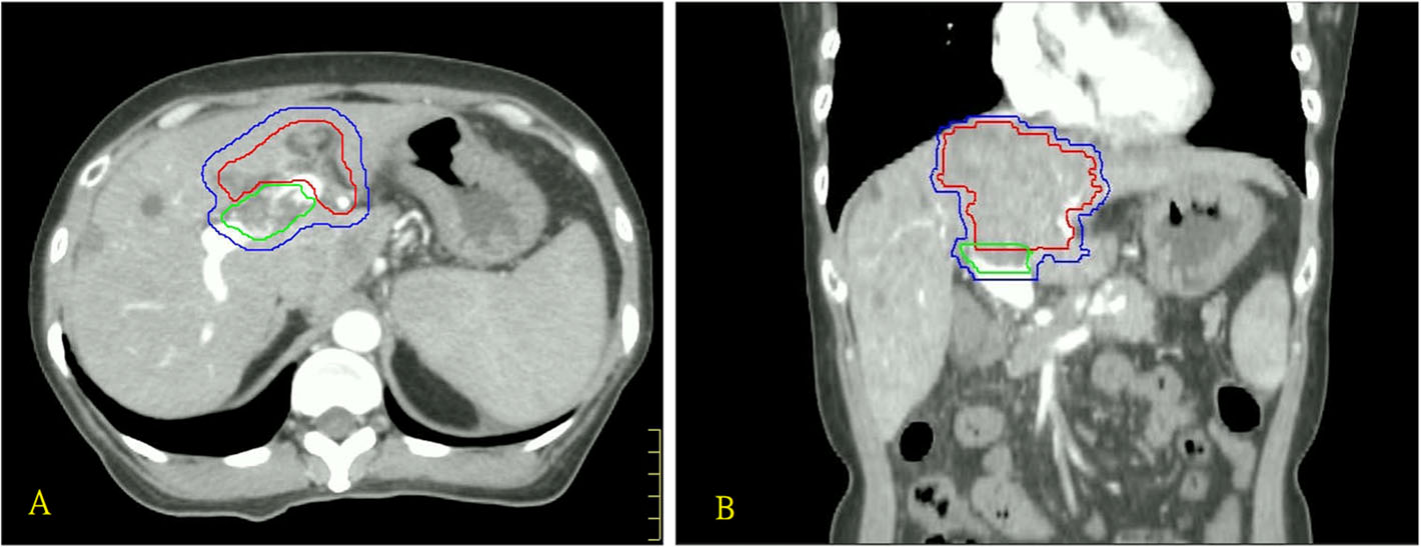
Example of target delineation in the axial (a) and coronal (b) view of computed tomography scans. The green, red, and blue lines represent detectable portal vein invasion, adjacent tumor, and clinical target volume, respectively
